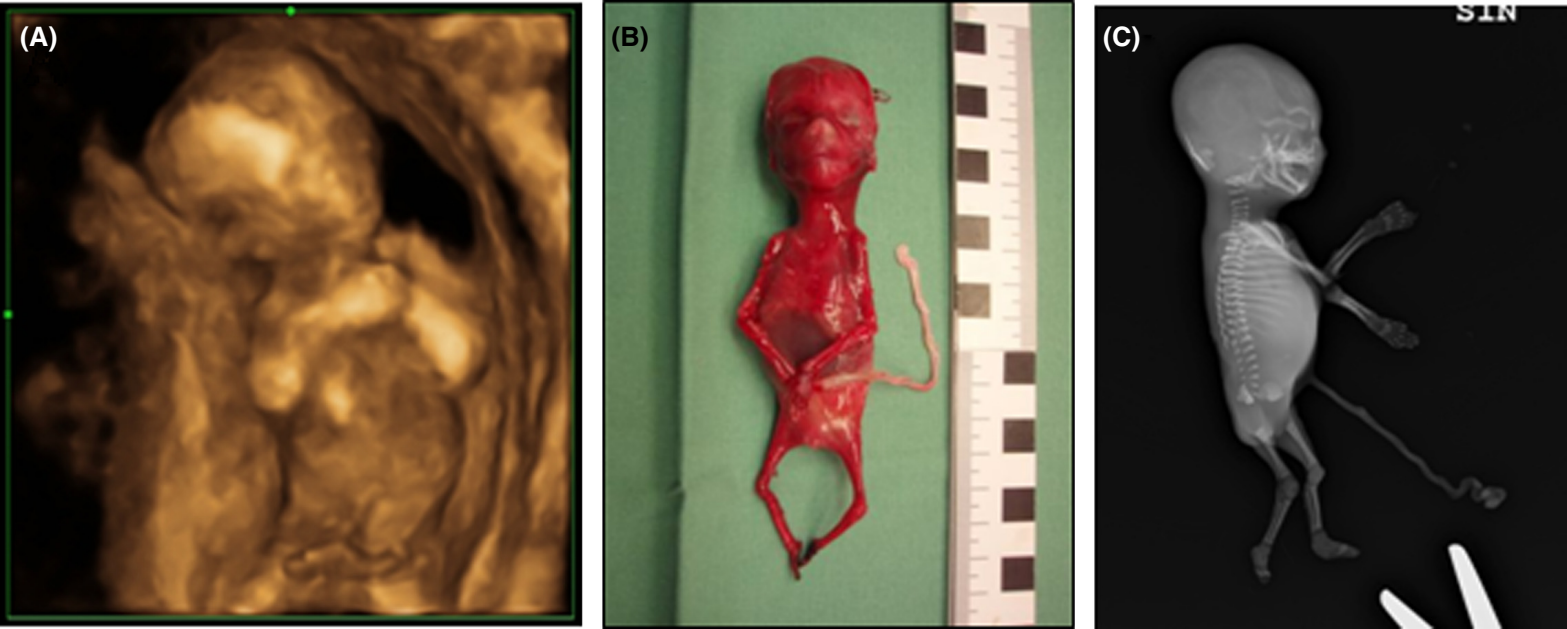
Prenatal appearance at 13 and 15 weeks gestation. (A) Ultrasound image showing the fetus with joint contractures of the upper limbs. (B) Abortus (15 weeks and 2 days) with severe hypoplasia of skeletal muscles, joint contractures, and craniofacial dysmorphism. (C) Radiograph of the fetus at 15 weeks and 2 days gestation.
